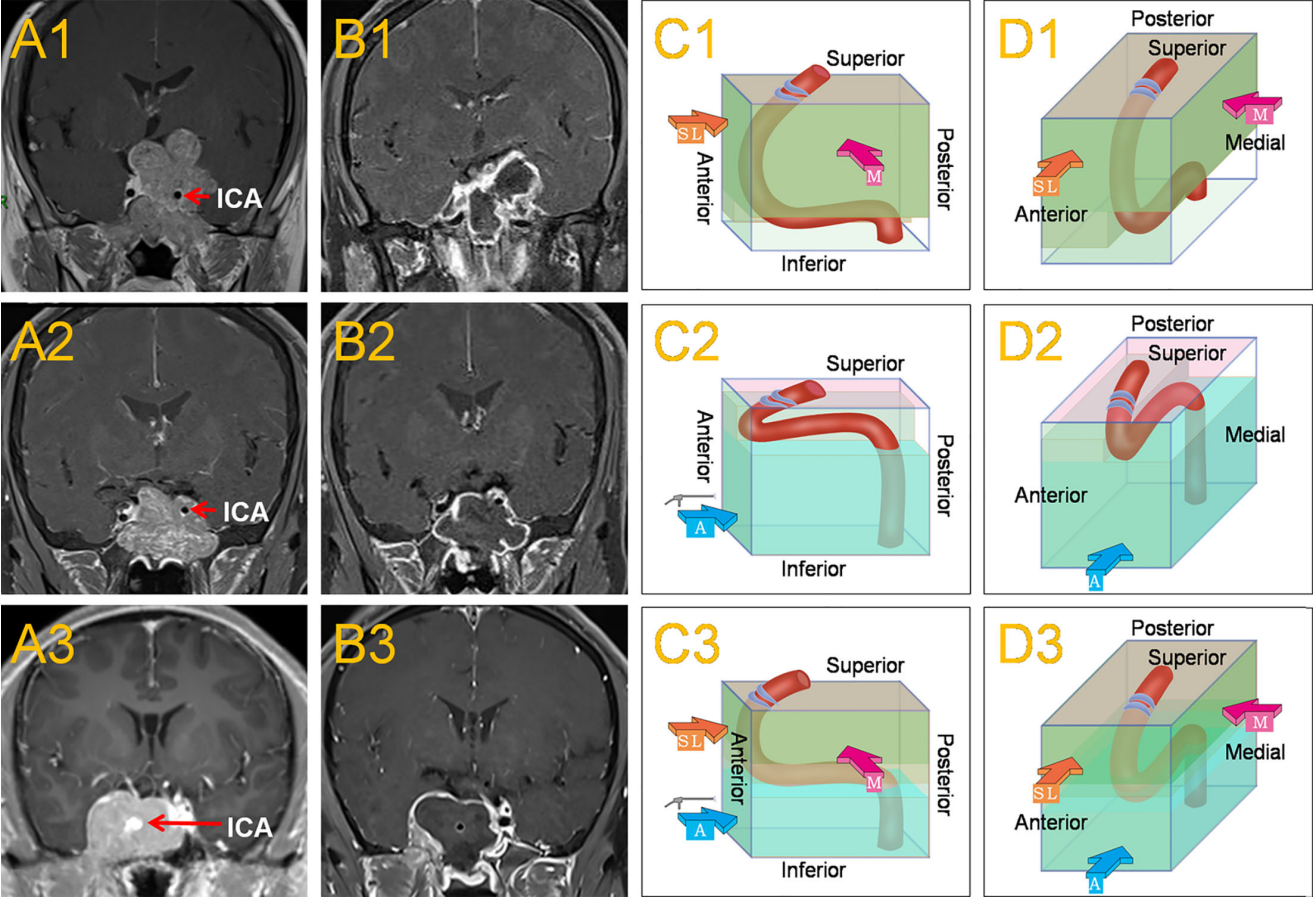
This shows the common location of the horizontal ICA in Knosp grade 4 PAs and the main surgical approaches. When the horizontal ICA was inferior to the tumor (A1), it caused the horizontal ICA to sink due to the oppression of the tumor, resulting in a larger posterosuperior compartment and a smaller anteroinferior compartment within the CS. The medial approach, often combined with the superior-lateral approach was mainly used to remove the tumor (C1, D1). Postoperative MRI revealed a subtotal resection of the tumor (B1).When the horizontal ICA was superior to the tumor (A2), it caused the horizontal ICA to be raised by the tumor, leading to a smaller posterosuperior compartment and a larger anteroinferior compartment within the CS. The anteroinferior approach was mainly used to remove the tumor (C2, D2). Postoperative MRI revealed a total resection of the tumor (B2). When the horizontal ICA was in the middle of the CS tumor (A3), which was a combination of the two conditions, then the medial, superior-lateral, and anteroinferior approaches could be used (C3, D3). Postoperative MRI revealed a total resection of the tumor (B3). The red, thick arrow ‘M’ represents the medial approach, the orange-yellow, thick arrow ‘SL’ represents the superior-lateral approach, and the blue, thick arrow ‘A’ represents the anteroinferior approach.
